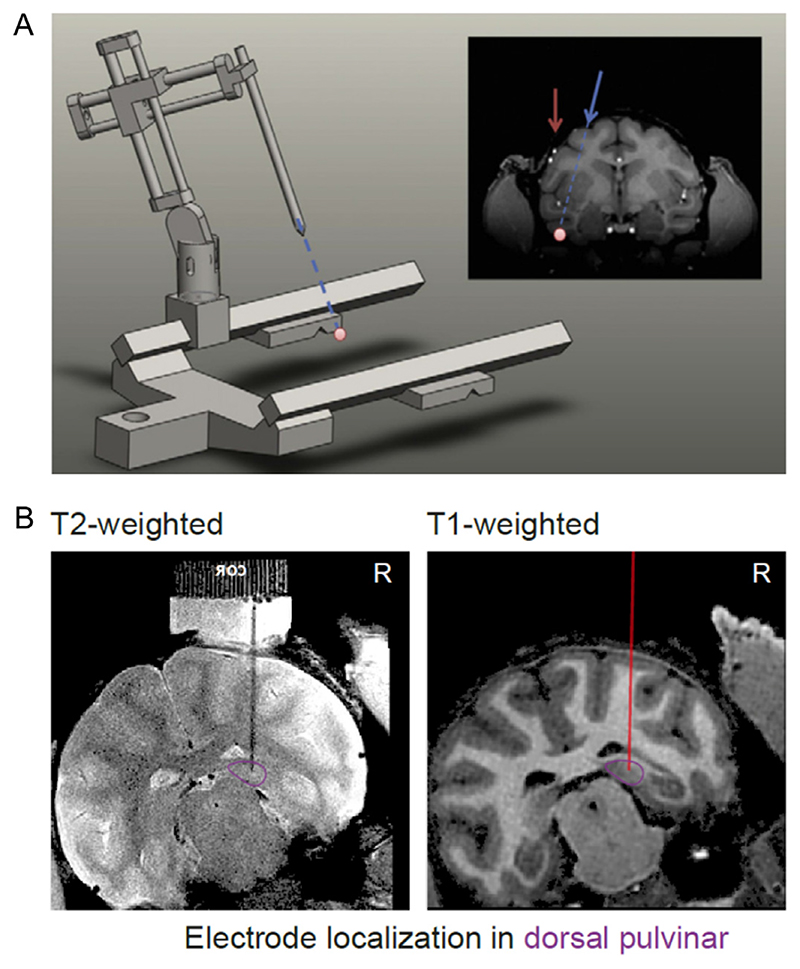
Targeting a part of the brain for perturbation. A) Targeting software (Planner) that allows the alignment of a stereotaxic manipulator with a target identified using MRI (pink dot). Reproduced from Ohayon and Tsao (2012). B) Pt-Ir electrodes can be localized using a T2-weighted scan and coregistered to a T1-weighted scan for more anatomical detail. Adapted from Dominguez-Vargas et al. (2017).
